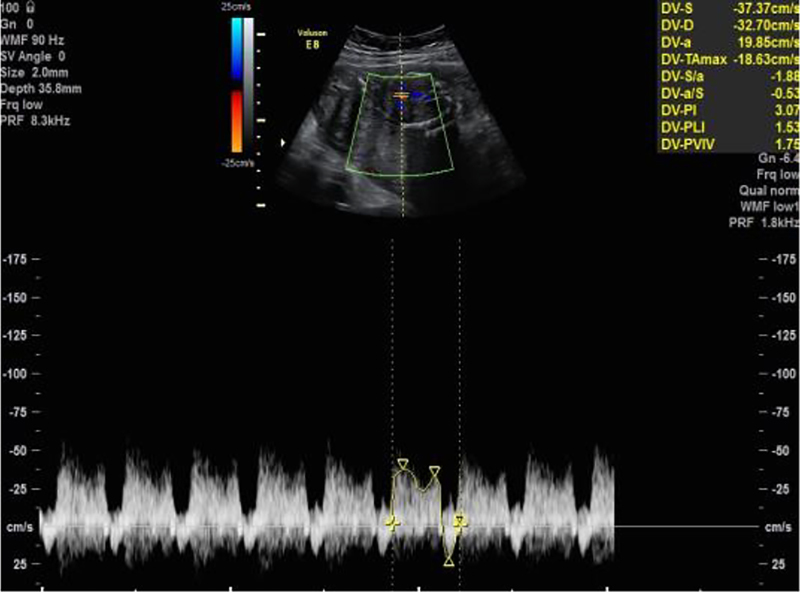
Ultrasound at 27 weeks showing a reversed a-wave in ductus venosus.
